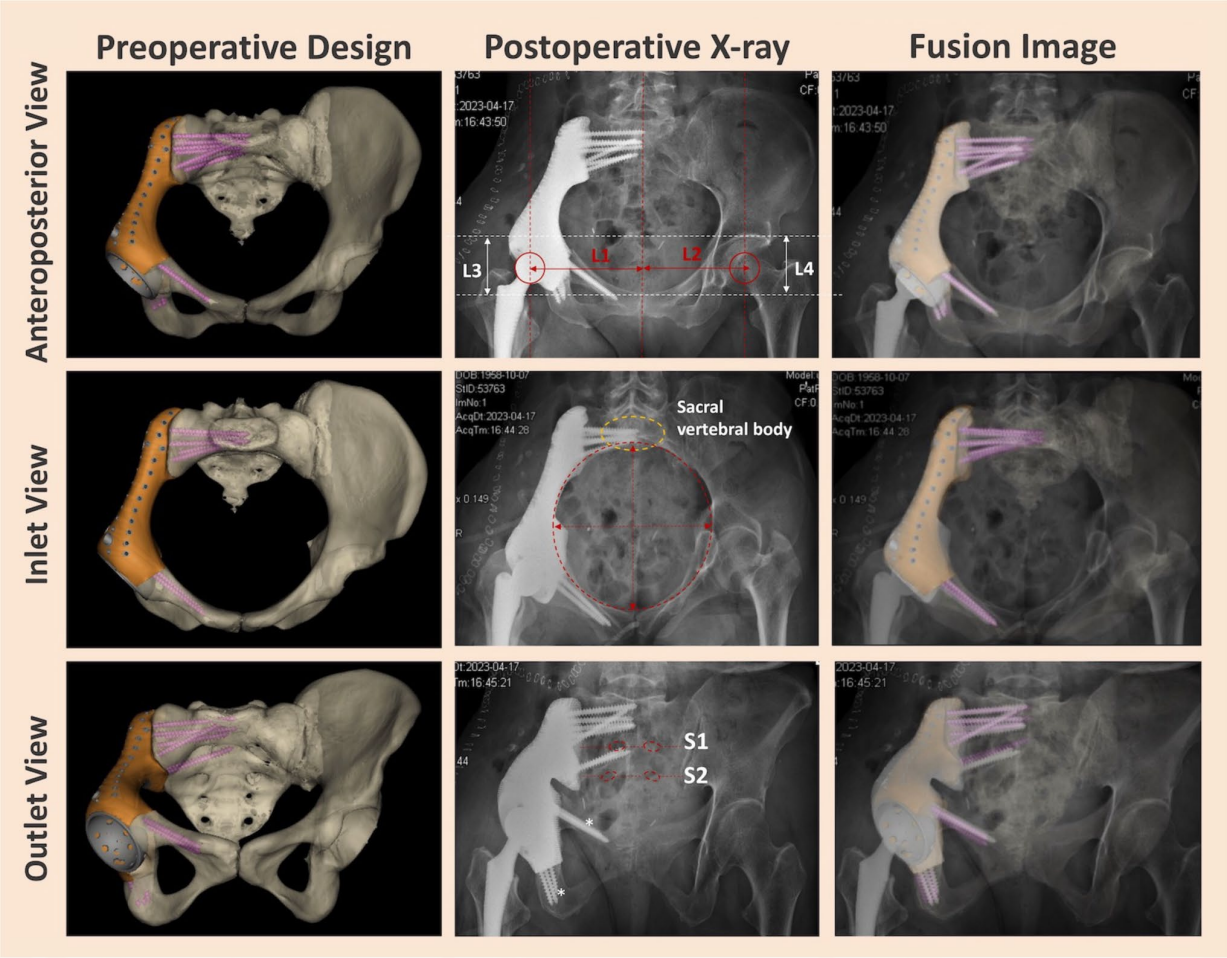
A typical case of 3D-printed custom hemipelvic prosthesis reconstruction: Postoperative pelvic X-ray evaluation confirms precise implantation of the 3D-printed custom hemipelvic prosthesis according to the preoperative plan, including accurate screw placement and prosthesis positioning. Anteroposterior views show the hip joint rotation centre (red circle) and femoral head eccentricity in both horizontal (L1 = L2) and vertical (L3 = L4) directions. Inlet views display sacroiliac joint screw placement, avoiding vertebral canal encroachment (yellow circle). Small pelvic ring reconstruction is evident (red dashed circle), and outlet views show accurate sacroiliac joint screw placement, taking care to avoid the S1/S2 sacral foramina (red dashed box). Ischium and pubic bone screws provide stability and support (Reprinted with permission from Hu et al. ©2024 Journal of Orthopaedic Surgery and Research [50])
